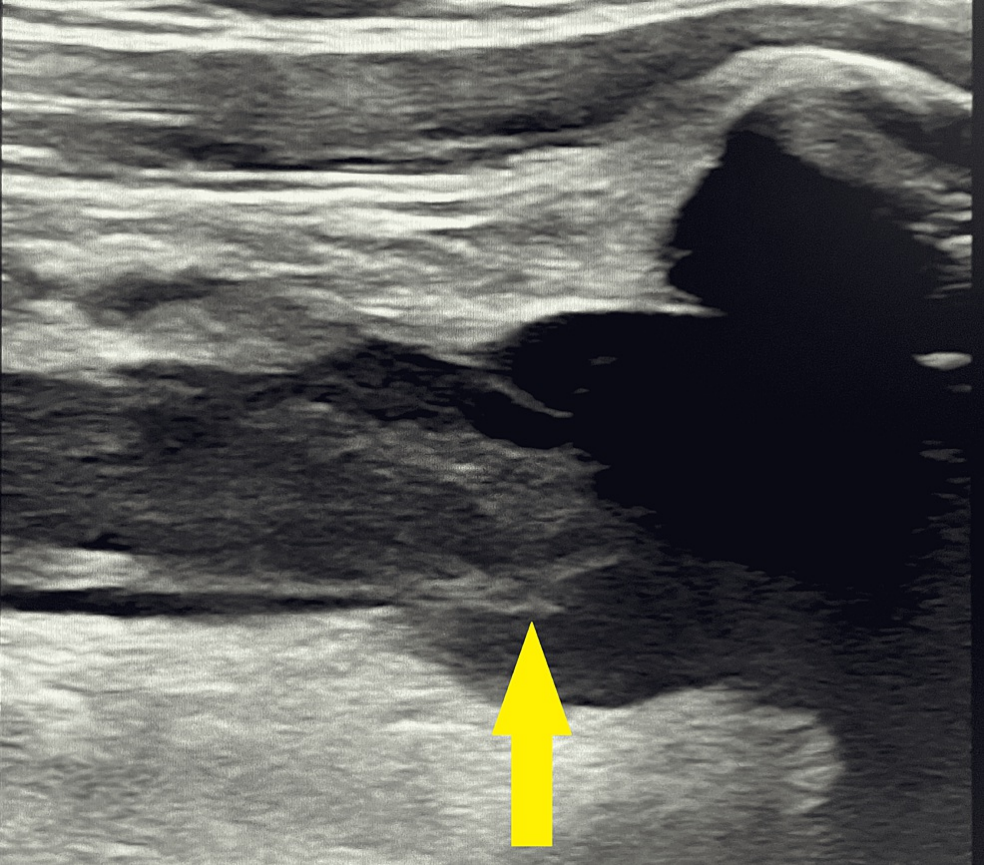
Ultrasound of right brachiocephalic vein with thrombus (arrow).
